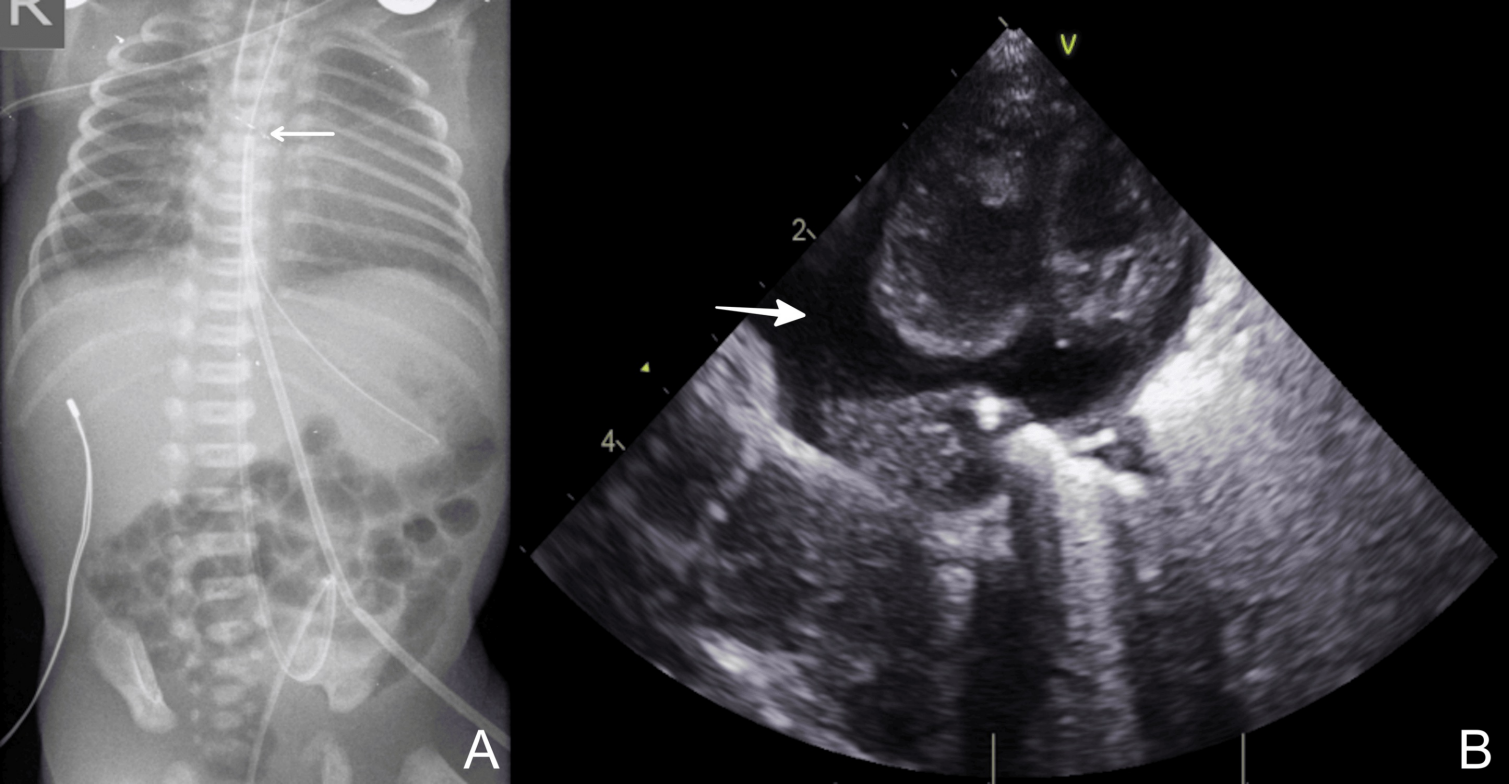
Case 3: (A) X-ray showing a high position of UVC (arrow). (B) Echocardiography showing the pericardial effusion (bold arrow). UVC: umbilical venous catheter
